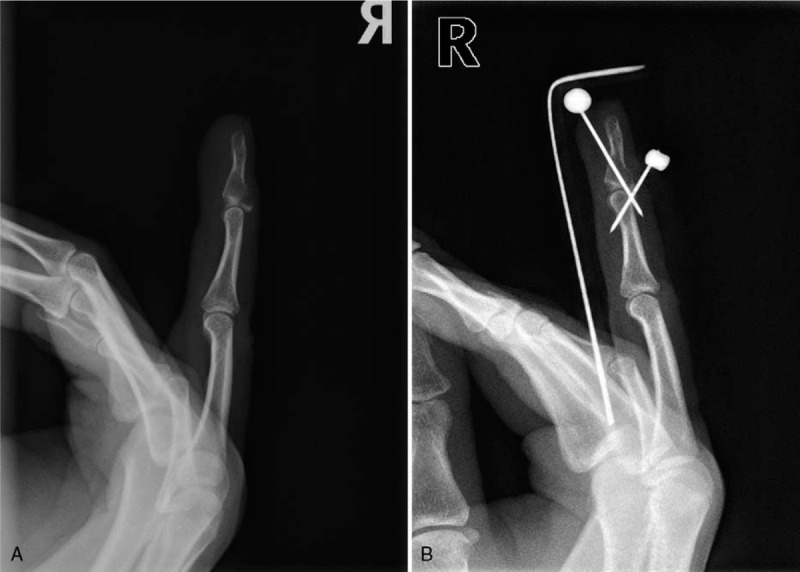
(A) Initial lateral plain radiographs of the patient's hand (B) Postoperative radiographs following extension block pinning with Kirschner wires.
